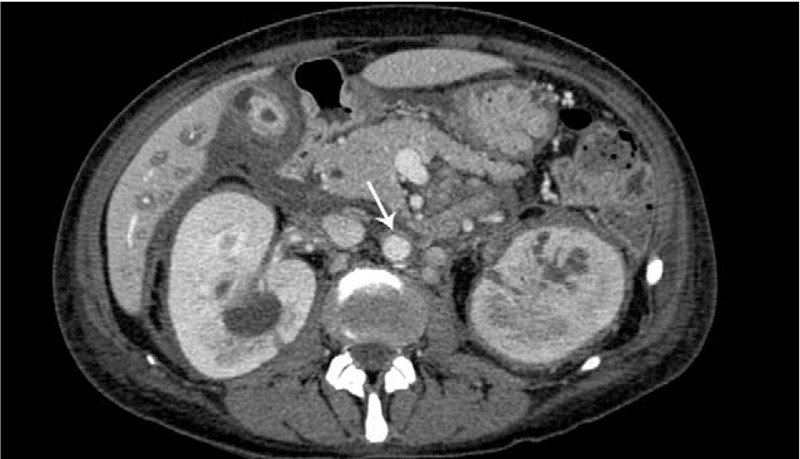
Axial contrast-enhanced abdominal computed tomography scan image in a 44-year-old woman, showing bilateral and symmetrical infiltration of the adrenals and renal sinuses with right hydronephrosis. Note also soft tissue rounding portal vein until liver hilus and intrahepatic branches. Periaortic concentric soft tissue, also known as “coated aorta,” is visible (arrow).
